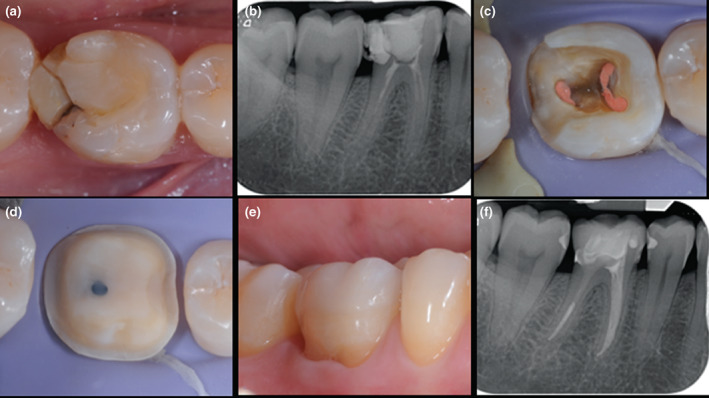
Lithium disilicate ceramic onlay (IPS e.max, Ivoclar Vivadent) placement following root canal retreatment of 36 (a, b) preoperative occlusal view and long‐cone periapical radiograph (c) cleaned and prepared dentine surface prior to deep margin elevation and core placement (d) completed core and onlay preparation (e, f) post‐treatment occlusal view and postoperative periapical radiograph.
